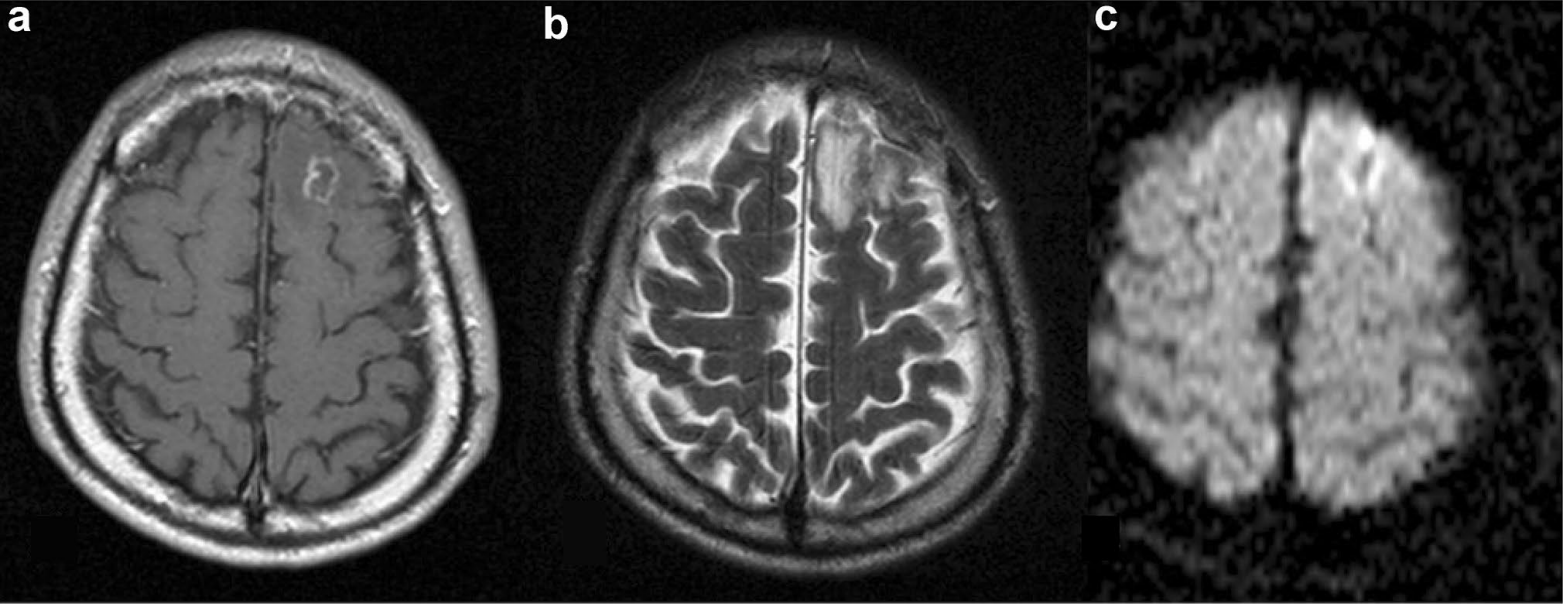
Ring-enhanced lesion in a patient with HIV-related primary CNS lymphoma. Contrast-enhanced T1-weighted image (a) shows a ring-enhanced lesion in the left frontal subcortical white matter. The mass shows a heterogeneous signal on T2-weighted image (b) with surrounding edema. On diffusion-weighted image (c), the lesion shows peripheral hyperintensity
